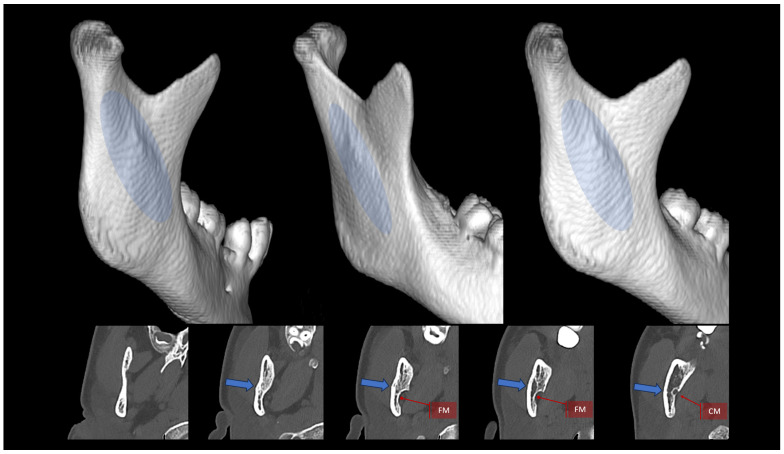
Appearance and location of the oblique augmentation of the condylar process and ramus of the mandible. Top: three-dimensional reconstructions created from a CT scan. Blue ellipse indicates the course of this lateral bony augmentation from the mandibular neck downward and anteriorly toward the ante-gonion notch. Bottom: horizontal cross sections. The blue arrow indicates the lateral augmentation. FM—foramen mandibulae; CM—canalis mandibulae. The radiological multiplanar reconstructions were performed using RadiAnt (www.radiantviewer.com/en; access date: 30 November 2024).
